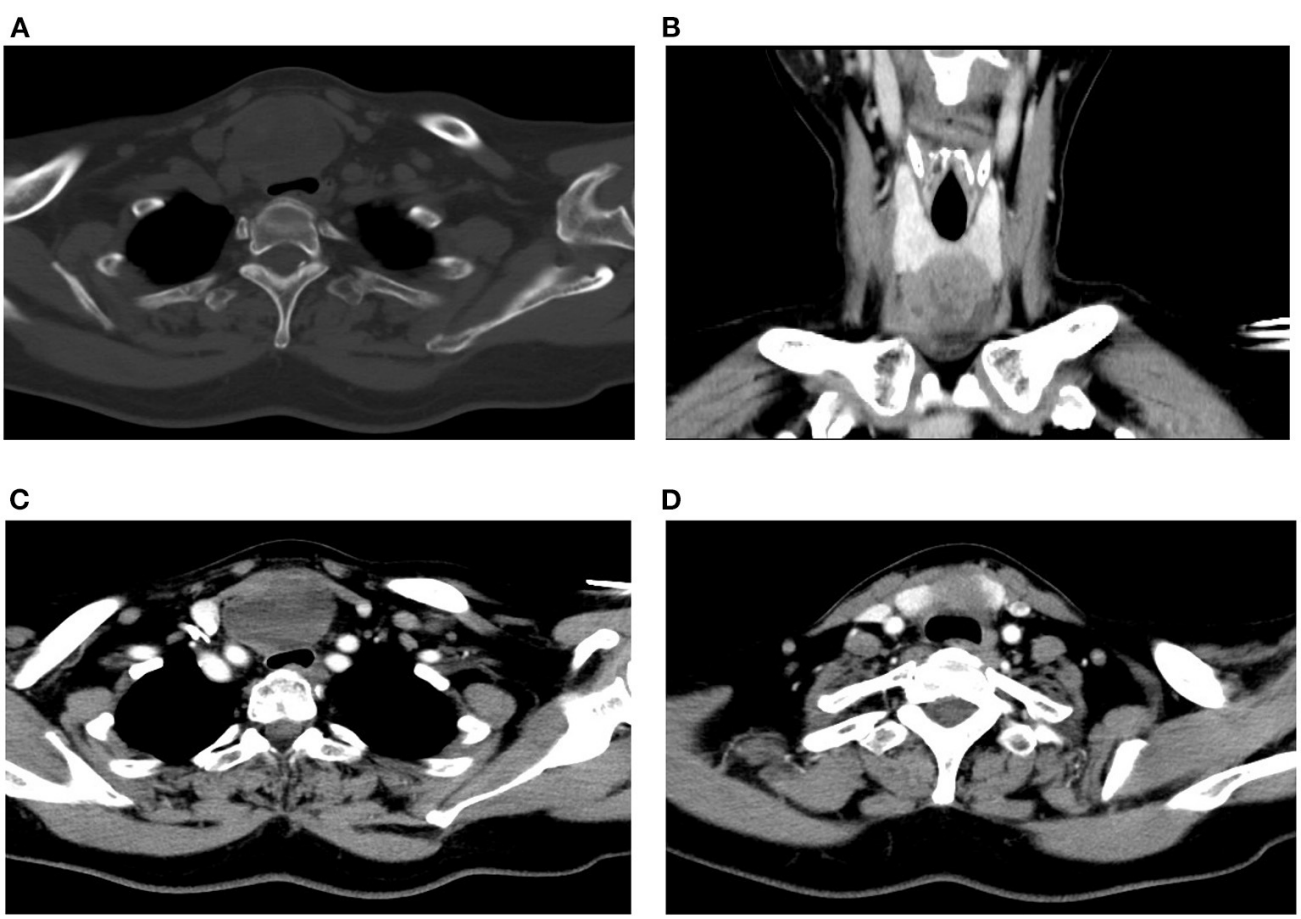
Preoperative CT scan of the patient. (A) Plain CT of the neck (horizontal plane). (B) CT scan of the neck (coronal plane). (C, D) Contrast-enhanced CT of the neck (horizontal plane).
